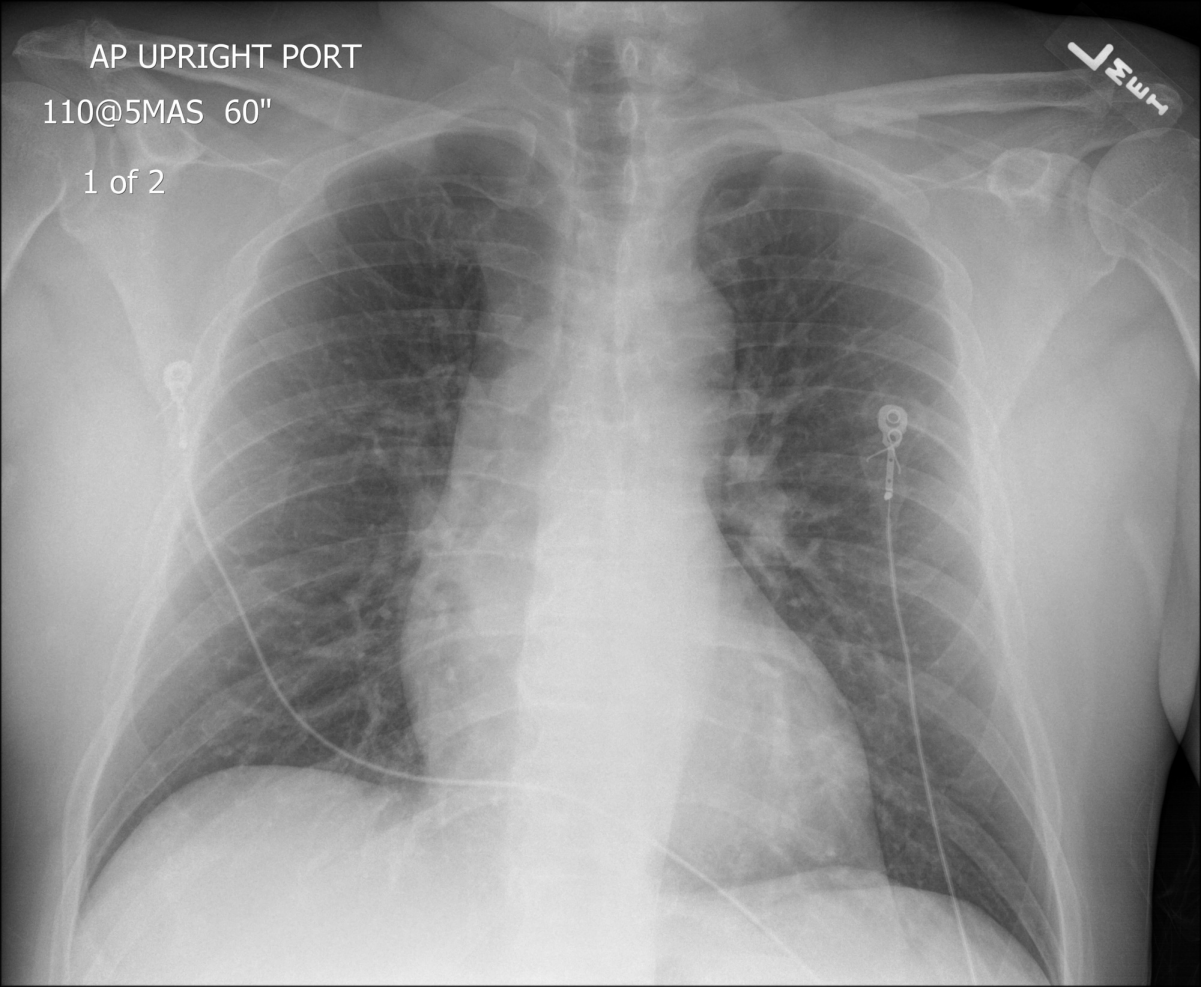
Chest X-ray. Portable chest X-ray, anterior-posterior orientation.
